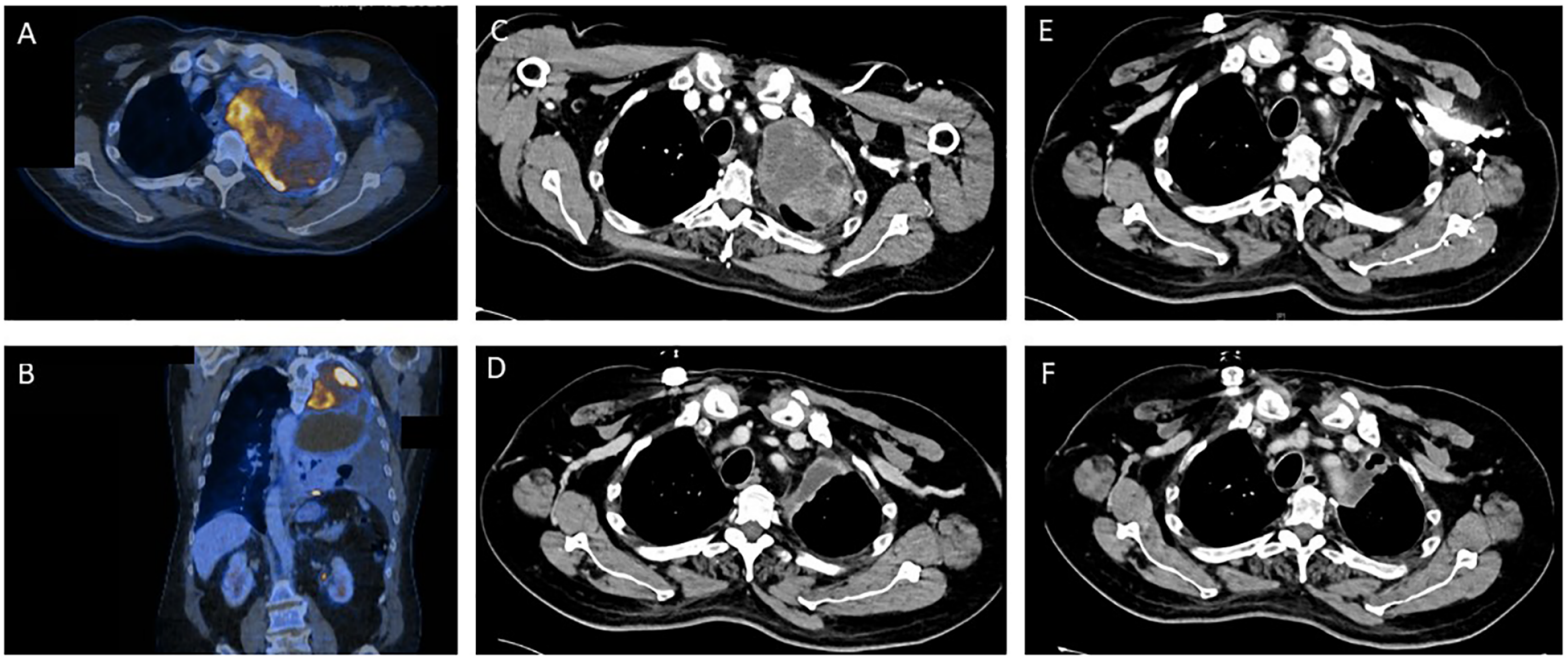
Tumor regression as depicted by sequential contrast enhanced computed tomography (CT) and positron emission tomography (PET) before and during the various stages of treatment for Ewing sarcoma. (A, B) Pre-therapeutic PET/CT imaging. (C) CT imaging prior to start of Ewing sarcoma chemotherapy. (D) CT Imaging after 2.5 cycles of induction chemotherapy. (E) CT Imaging after induction chemotherapy. (F) Post-operative CT imaging.
